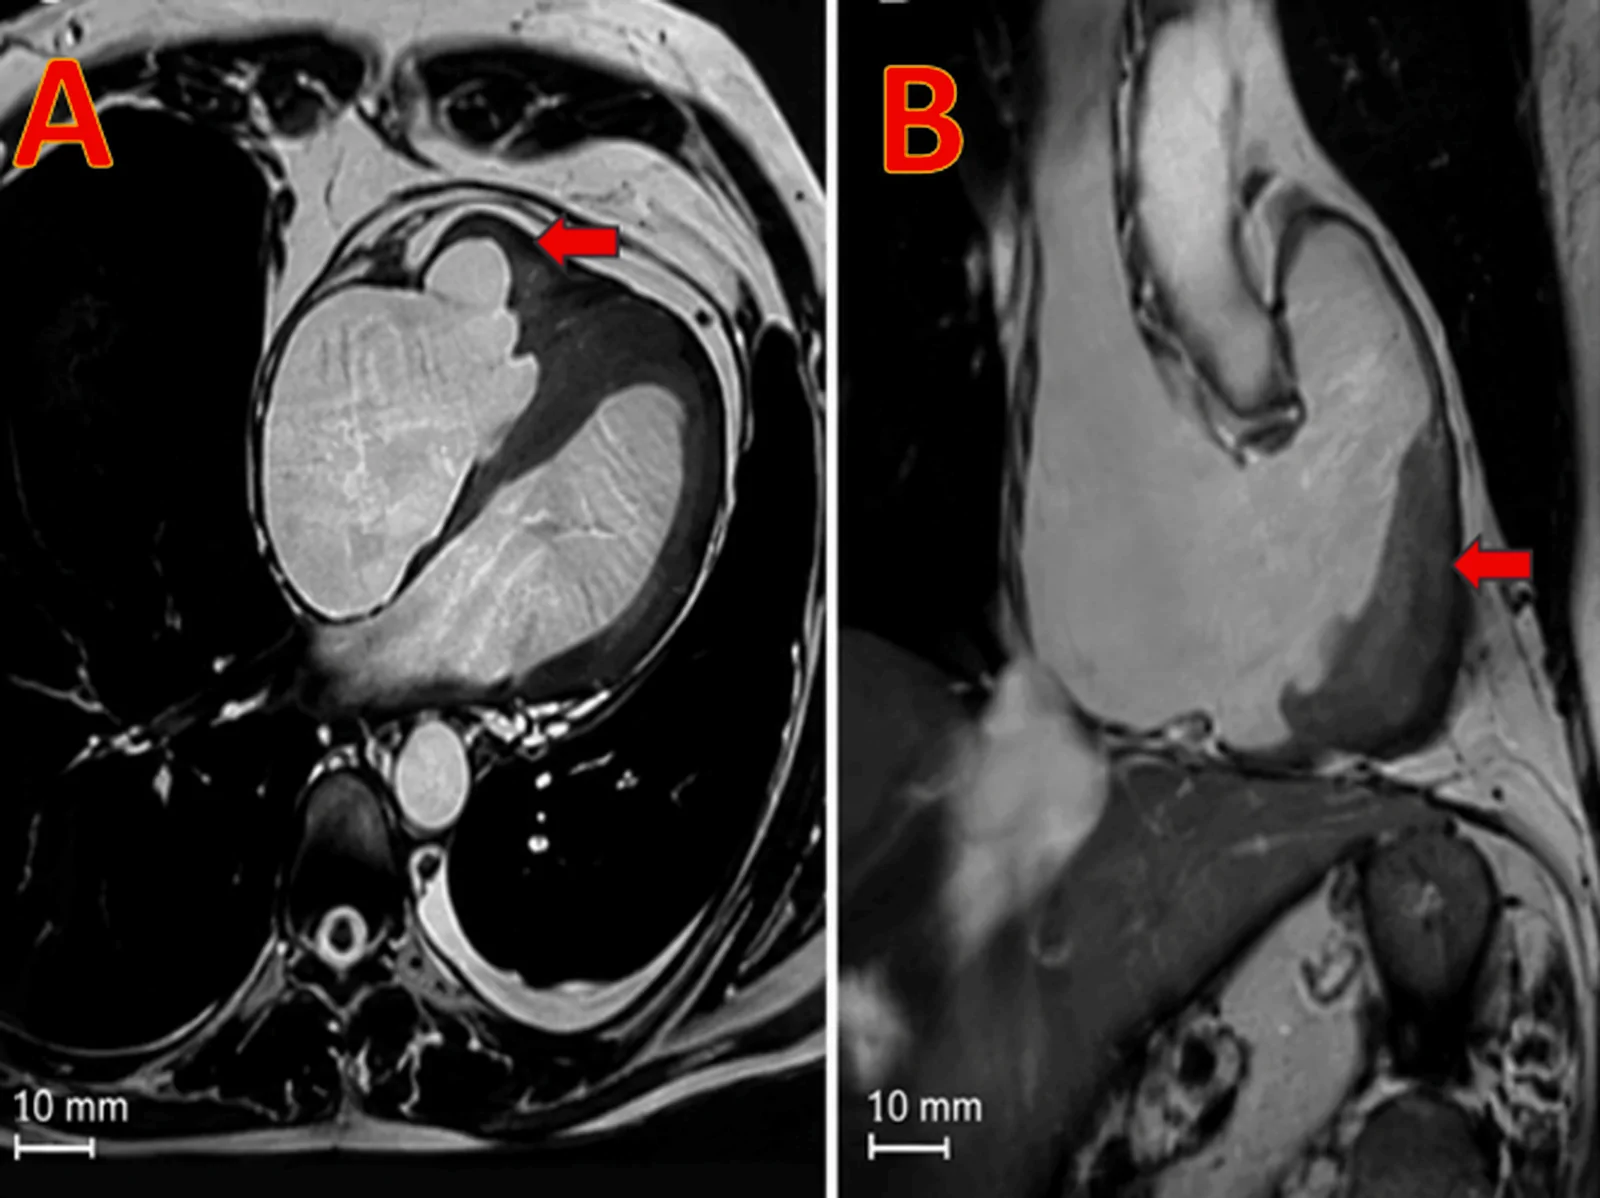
Cine steady-state free precession CMR images demonstrating isolated right ventricular endomyocardial fibrosis. (A) Apical four-chamber view and (B) right ventricular inflow-outflow view demonstrating extensive fibrotic obliteration of the right ventricular apex and inflow tract (red arrows), resulting in partial obliteration and severe reduction of the right ventricular cavity, consistent with advanced right ventricular endomyocardial fibrosis. CMR, cardiac magnetic resonance
